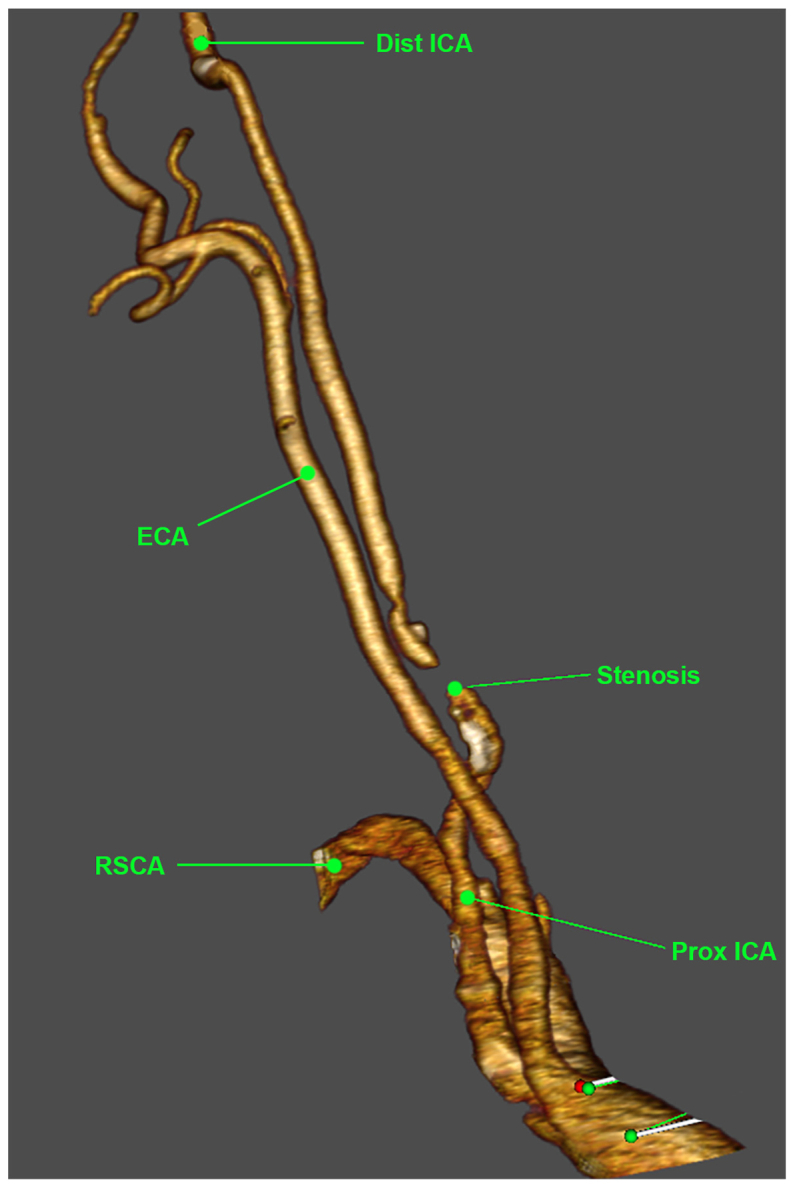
Preoperative three-dimensional chest computed tomography angiography. Frontal view of three-dimensional reconstruction of preoperative chest computed tomography angiography is shown with relevant structures labeled. The area of stenosis was approximately 3 to 4 cm from the anomalous origin of the right internal carotid artery (*ICA*) at the brachiocephalic artery. *Dist ICA*, Distal internal carotid artery; *ECA*, external carotid artery; *Prox ICA*, proximal internal carotid artery; *RSCA*, right subclavian artery.
